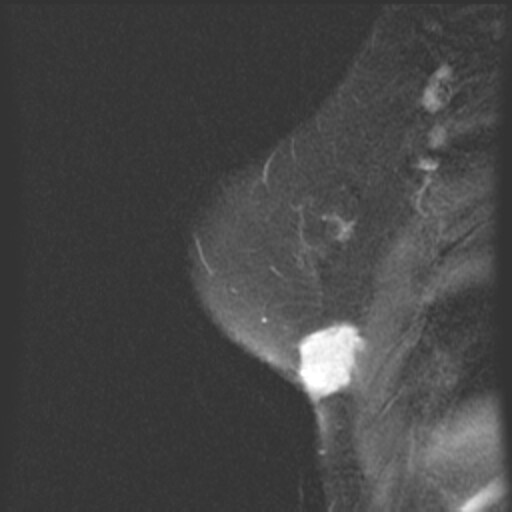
Magnetic resonance imaging contrast enhanced T-1 fat suppression sagittal view.
